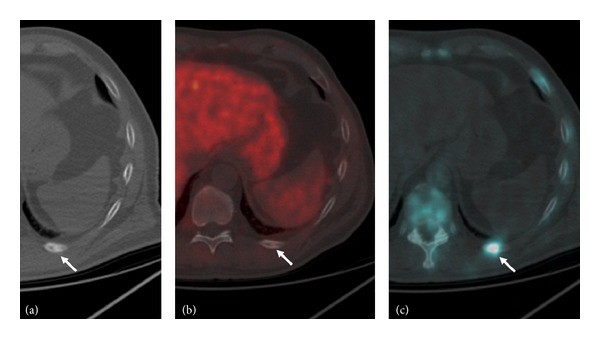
82-year-old man with a bone metastasis in the posterior arch of the left 10th rib. The lesion (arrow) is not definitely appreciable on the CeCT axial image (a) and is not detectable on the ^18^F-Choline-PET axial scan (b). On the ^18^F-Fluoride-PET/CT axial image (c) the skeletal metastasis is well detectable.
